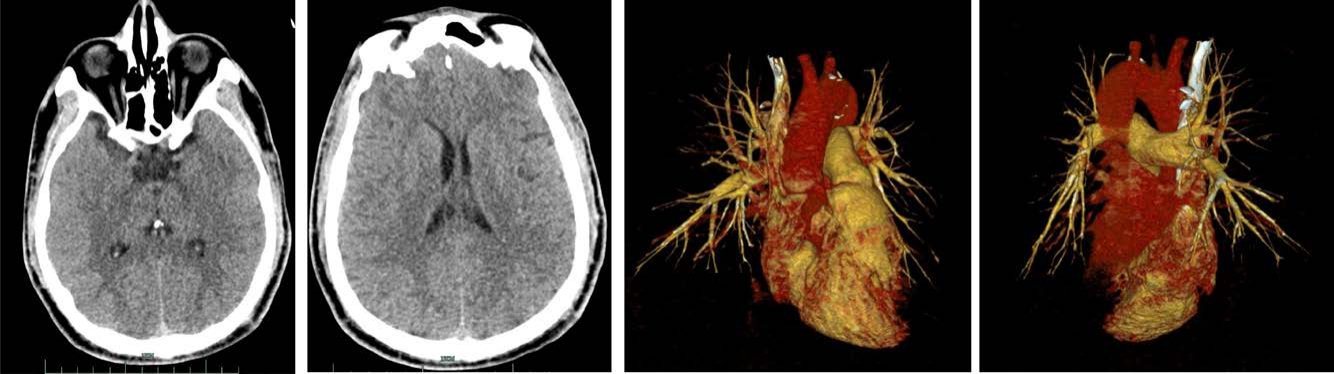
Craniocerebral CT: cerebral tissue has no obvious signs of hemorrhage and infarction. Pulmonary artery CTA: the main trunk and branches of both superior and inferior pulmonary arteries were well displayed, running naturally, no obvious dilatation and stenosis, and no obvious filling defect in the official cavity. The position of the heart with the aorta, pulmonary artery, and pulmonary vein was normal. CT = computed tomography, CTA = CT angiography.
